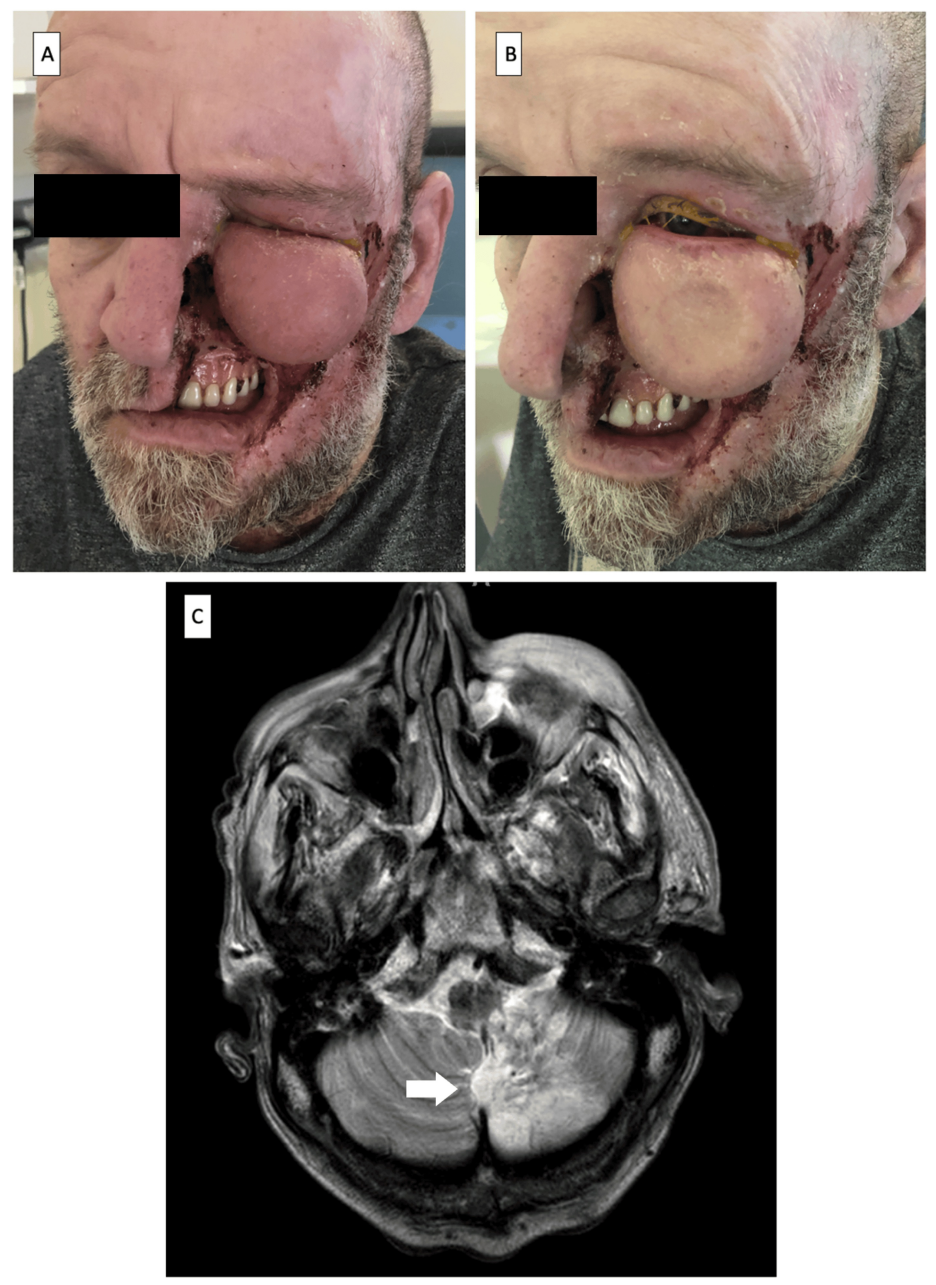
Images of case 2 (A,B) Mid-facial ulcerations in the left maxillary nerve dermatome, extensive soft tissue loss, absent left ala nasi but sparing of the tip of the nose, and severe left lower lid pitting lymphoedema. (C) MRI T2/FLAIR sequence showed established left PICA territory infarct and left malar soft tissue edema.
